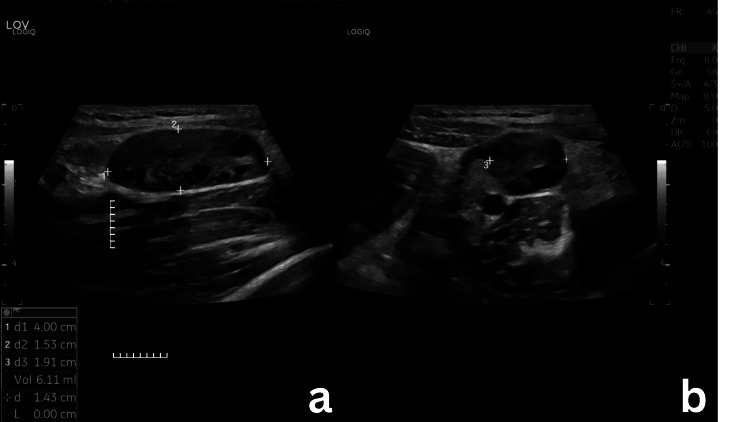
Follow-up ultrasound examination six months after oophoropexy (a) Follow-up ultrasound image demonstrating preserved morphology of the left ovary with normal follicular distribution. (b) Ultrasound measurements confirming decreased ovarian volume (6.11 mL) and normal postoperative appearance.
